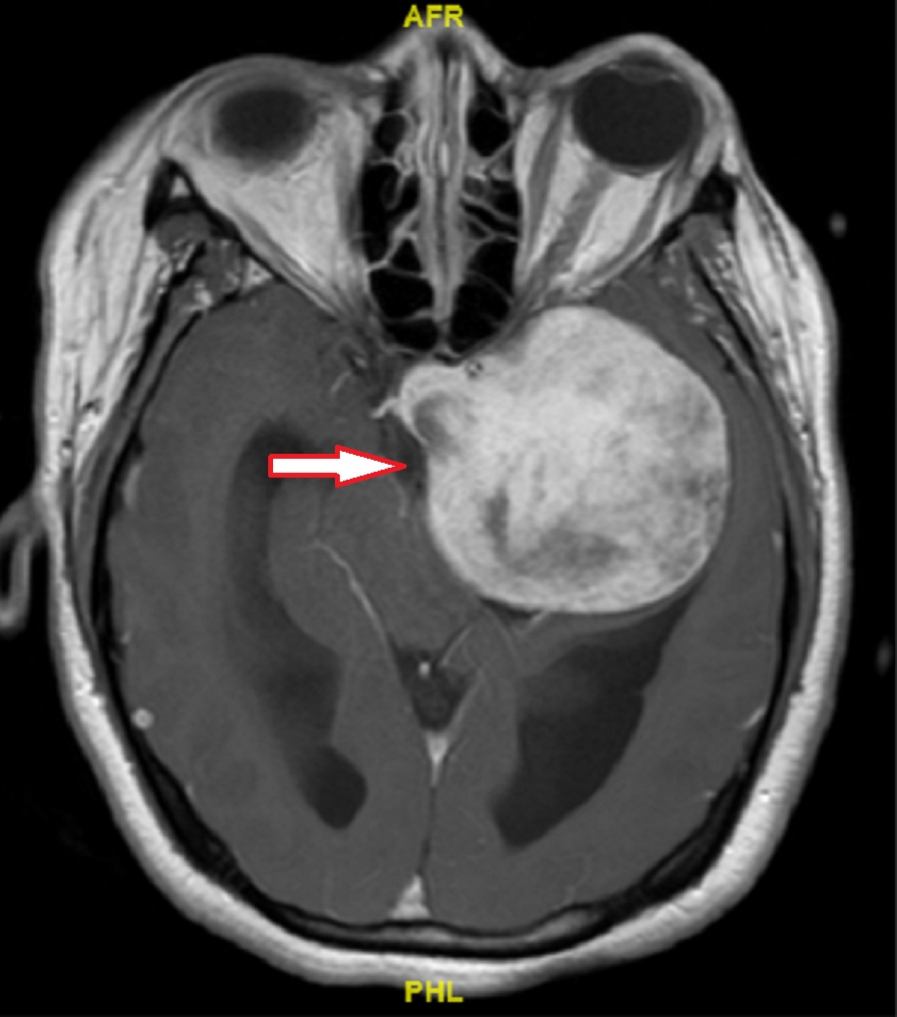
Initial T1 Contrast-enhanced Magnetic Resonance Imaging Axial view of tumour (arrow)
